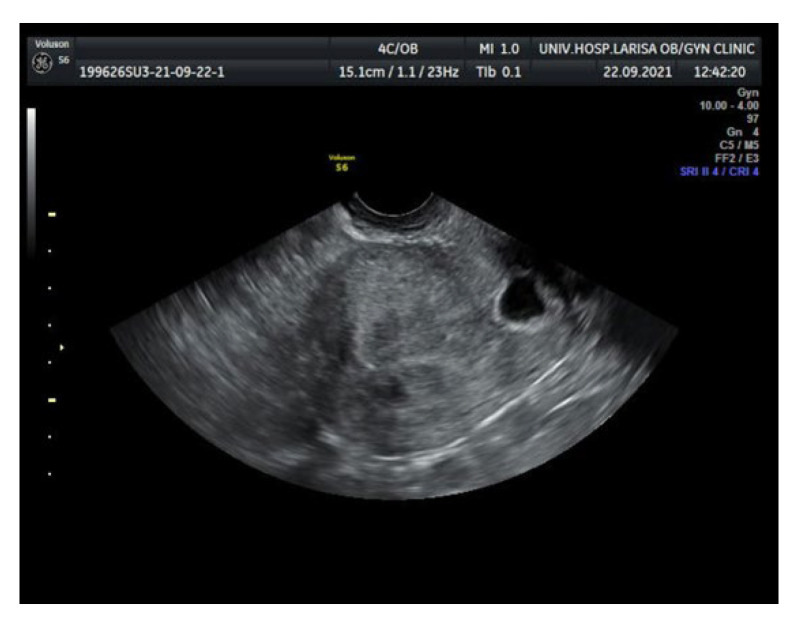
Transvaginal ultrasound 7 days after methotrexate administration.
